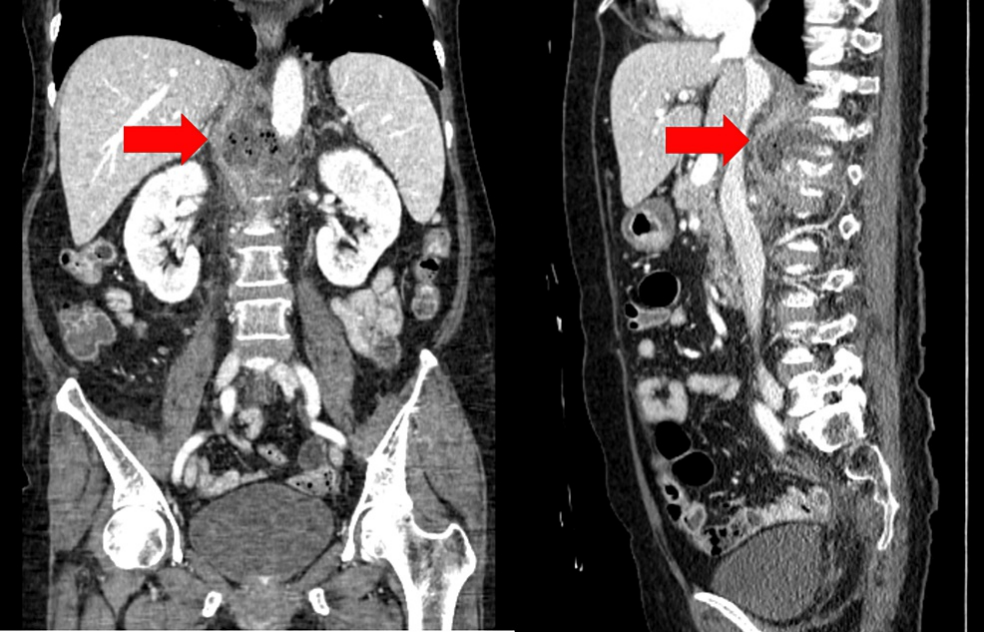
Abdominal and pelvic computed tomography coronal, sagittal view: abscess pocket from the T11 to L2 level (red arrows).
